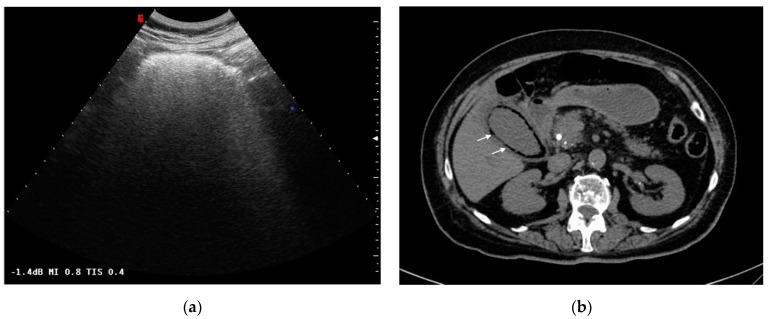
Emphysematous cholecystitis: (a) Ultrasonography: diffuse hyperechogenicity due to extended reverberation artifact with poor gallbladder lumen resolution. (b) CT findings: gallbladder wall thickening with intramural air (white arrows). CT was able to detect and characterize the disease.
